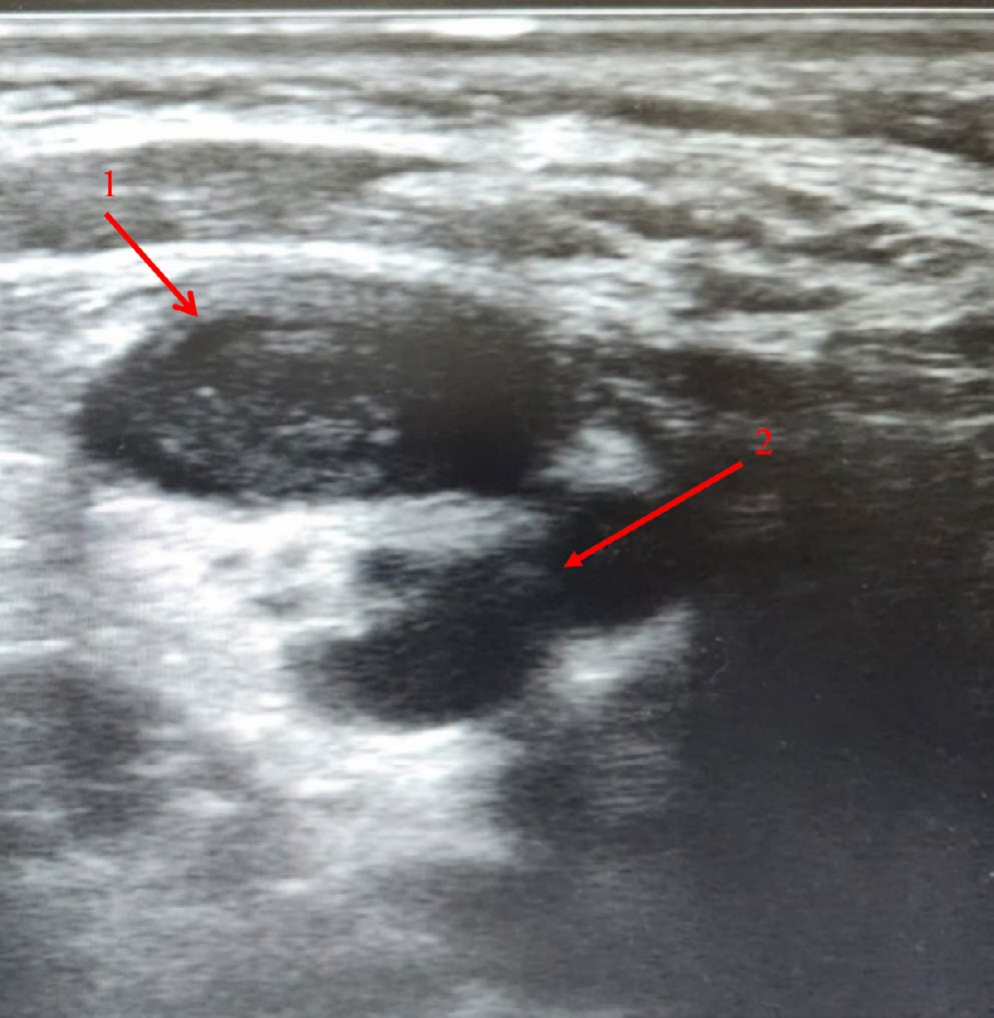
Cervical ultrasonography demonstrating a heterogeneous hypoechoic collection with internal echoes (arrow 1) adjacent to a dilated right internal jugular vein containing heterogeneous echogenic intraluminal material without Doppler flow (arrow 2), consistent with a cervical abscess (approximately 21 mL) and associated internal jugular vein thrombosis.
